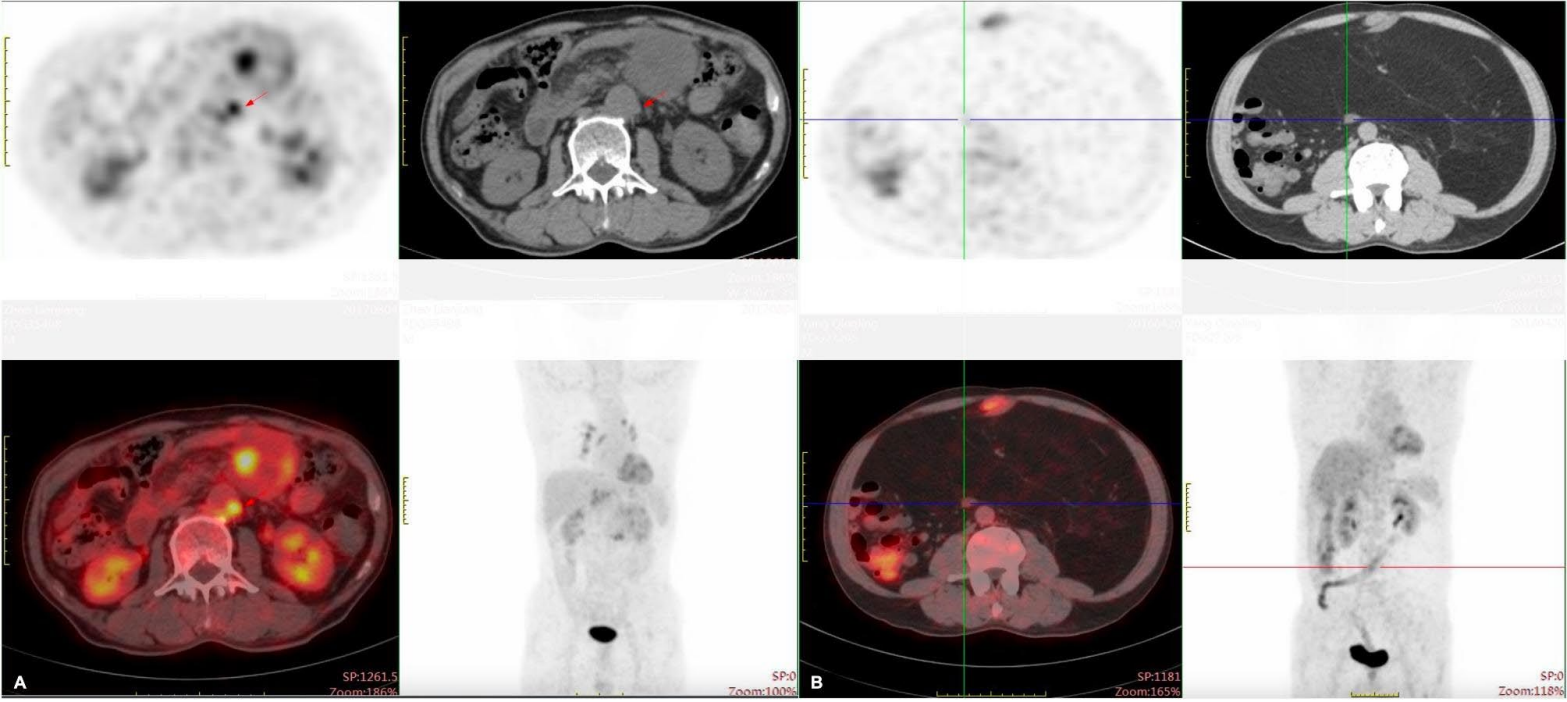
A shows a patient with non-recurrent PLPS with 9 cm diameter, 6.1 SUVmax, 618.2 MTV, and 1537 TLG in our cohort. The patient had 18 months DFS and 34 months OS. B shows a patient with non-recurrent WDLPS with 45 cm diameter, 1.4 SUVmax, 0 MTV, and 0 TLG in our cohort. The patient does not experience recurrence until the last follow-up, and its OS is 84 months
